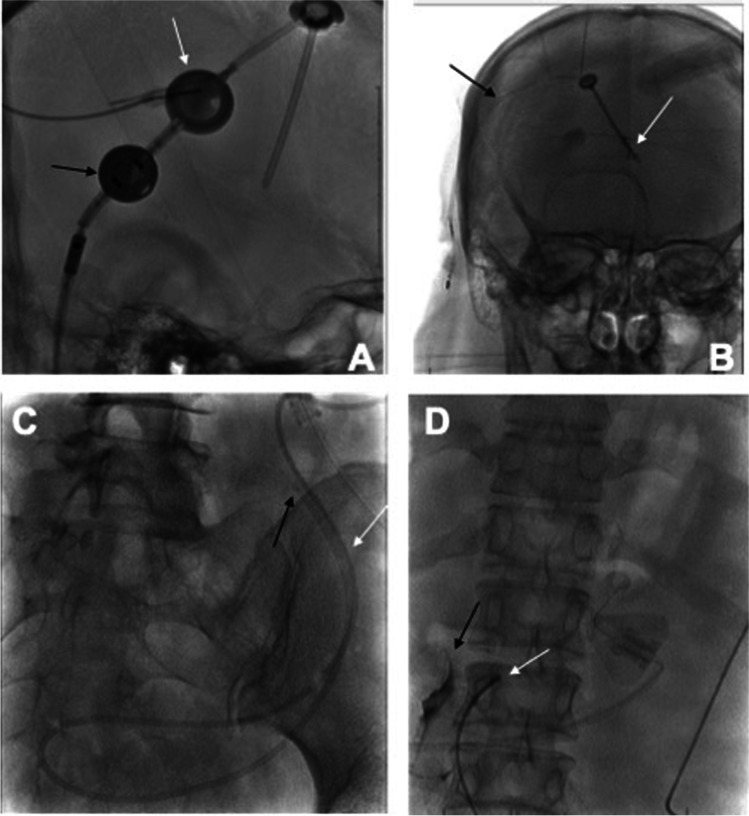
Clinical examples of radiographic imaging of shunt dysfunction (description in Table 1)
